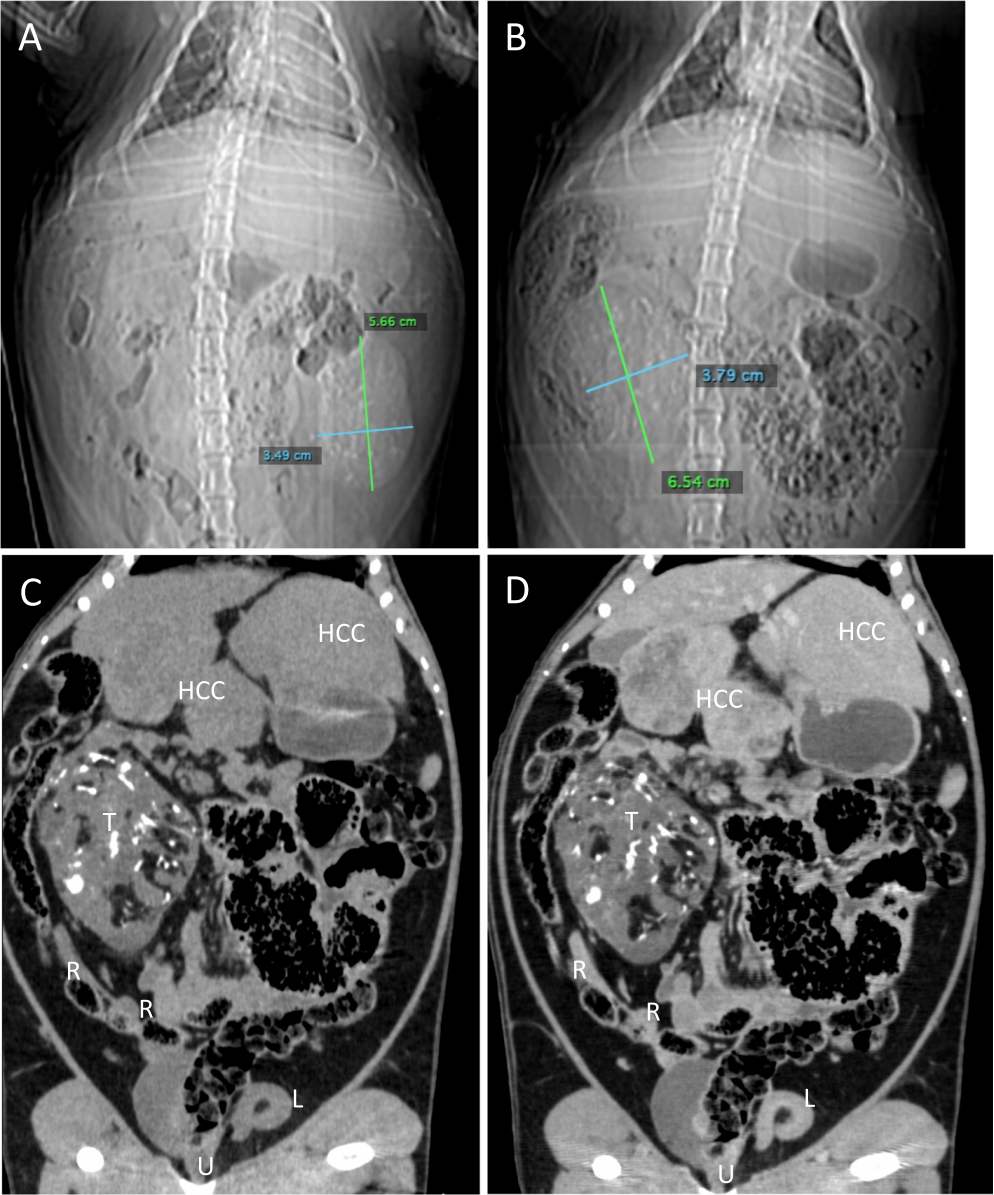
Computed Tomography. a Initial CT scout image and b CT scout image five weeks later showed a large mass with calcifications in the left abdomen on the initial scan and in the right abdomen five weeks later. Coronal reconstructions of c non-contrast scan and d 1-min delayed scan after contrast show the teratoma (T) as predominantly soft tissue density with areas of fat and dense calcification. The right uterine horn (R) is partially shown but could be traced from the body of the uterus (U) to the teratoma while the left uterine horn (L) is coiled in the caudal abdomen or pelvis. Two hepatocellular carcinomas (HCC) are shown: a 5.8 cm HCC in the left medial lobe with heterogenous enhancement and necrotic regions on this delayed image and a 3.3 cm HCC in the left lateral lobe
